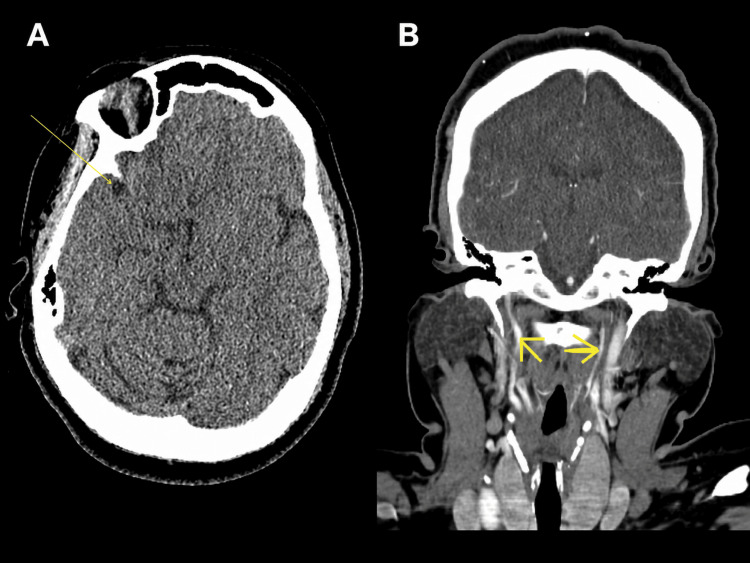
CT head A) CT demonstrates a 6 mm, well-circumscribed hypodense focus within the right frontal lobe of indeterminate etiology as depicted by the arrow. Given the patient’s age, a demyelinating process remained within the differential diagnosis; B) Coronal maximum intensity projection (MIP) image demonstrates left greater than right hypoplasia of the extracranial internal carotid arteries at the level of the upper cervical spine (bilateral arrows).
